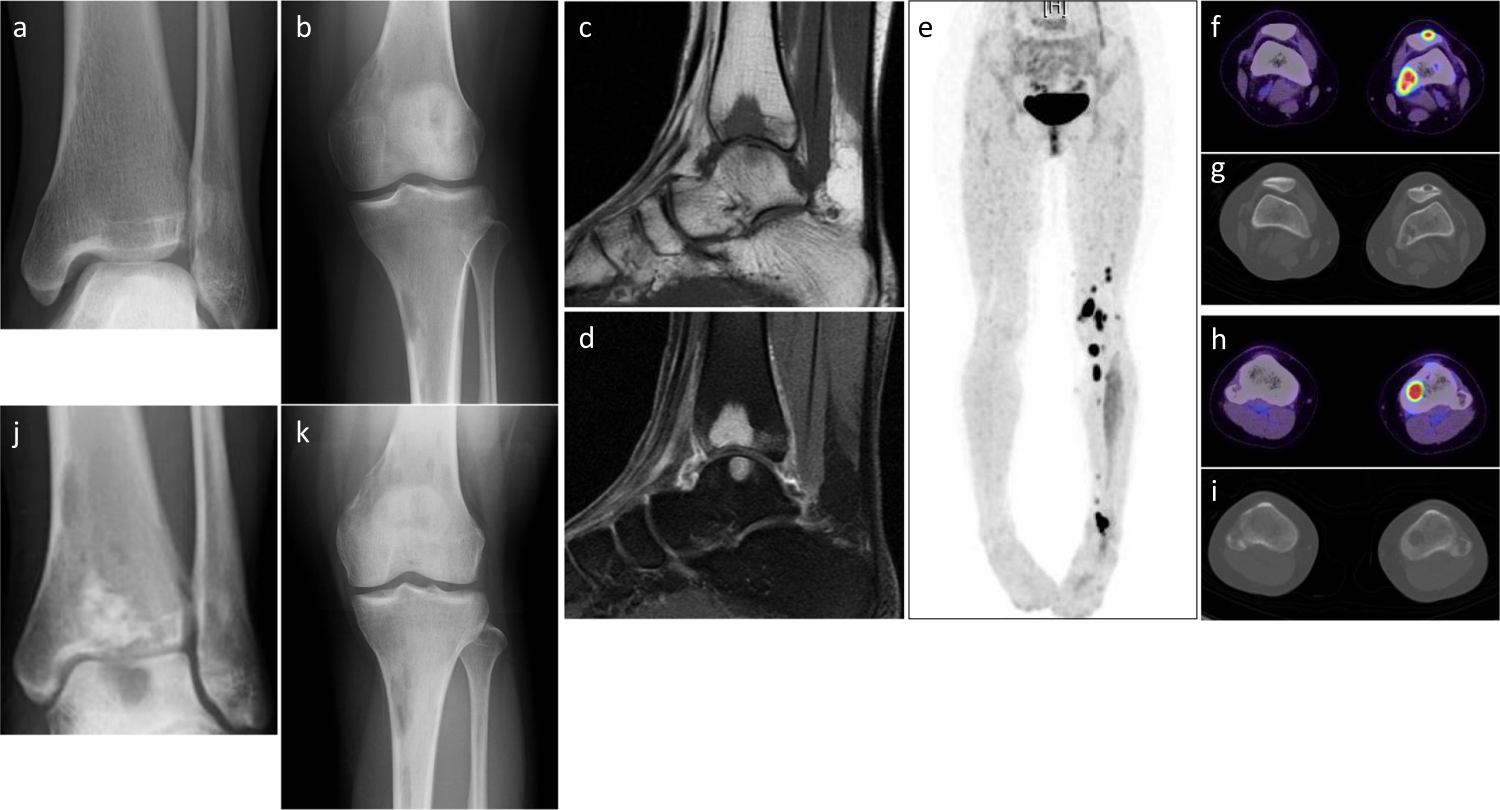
Imaging findings at presentation. a and b Plain X-ray findings. Multifocal ill-circumscribed lytic lesions were seen in the left femur, patella, tibia, and talus. c and d Sagittal magnetic resonance imaging findings of the left ankle. The multifocal lesions showed c homogeneous low intensity on T1-weighted images and d homogeneous enhancement after gadolinium administration. e-i Fluorodeoxyglucose (FDG)-positron emission tomography/computed tomography showed multifocal FDG-avid lesions in the bones of the left lower extremity with a maximum standardized uptake value of 15.95. No lesions were found in the soft tissue. j and k Rapid progression after curettage and artificial bone grafting of the lesion at the distal end of the left tibia was observed. Each ill-circumscribed lytic lesion in the left extremity increased in size over 1 month
